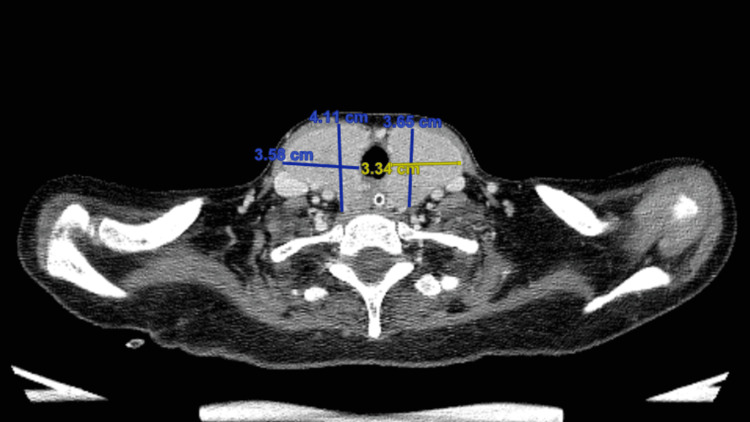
Neck CT (coronal view). The yellow and blue lines delineate the size of the thyroid gland. CT: computed tomography
